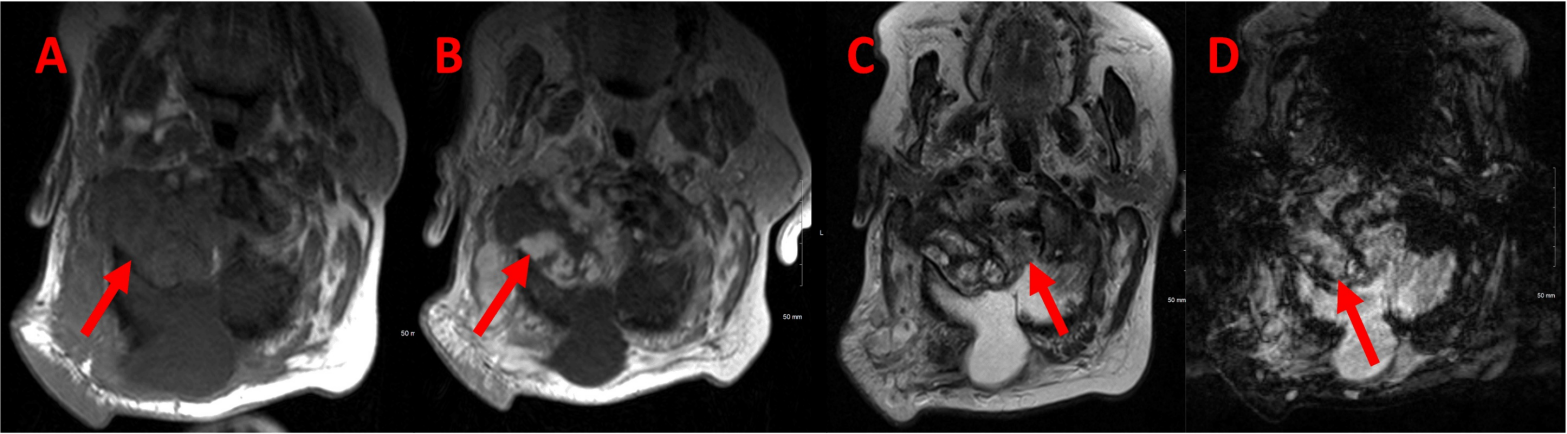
Axial magnetic resonance imaging of the patient's head - pre-treatment. (A) T1 (pre-contrast): The intermediate signal thrombus within the aneurysm is relatively innocuous on T1 (red arrow). (B) T1 (post-contrast): Post-contrast images demonstrate central mass-like enhancement of the aneurysm (red arrow). (C) T2: Mixed signal within the aneurysm, which displaces and compresses the cervicomedullary junction (red arrow) to the left. (D) Susceptibility weighted imaging (SWI): Susceptibility artifact is seen outlining the aneurysm wall (red arrow), another clue to the vascular nature of the pathology.
